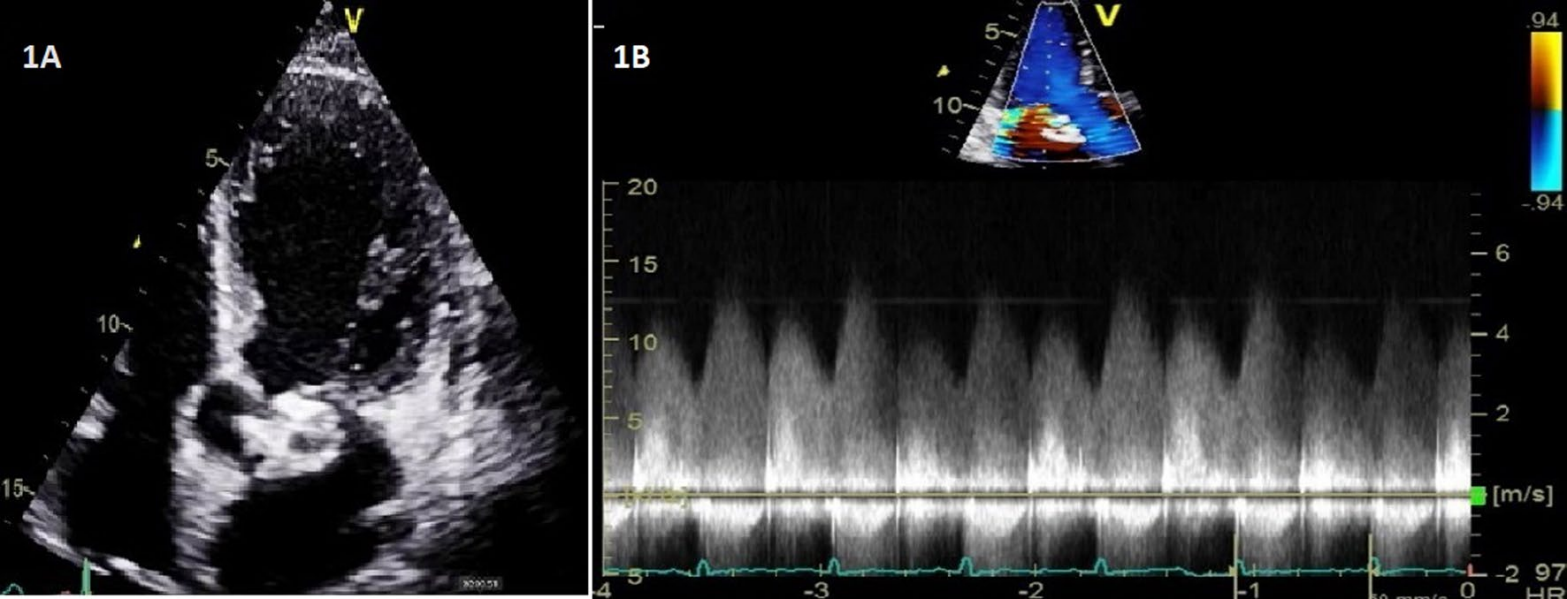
Transthoracic echocardiogram. A Apical five-chamber view on transthoracic echo showing vegetations above the aortic valve and an echo-free space in mitral-aortic intervalvular fibrosa consist with abscess. B Continuous-wave (CW) Doppler showing continuous flow due to fistula formation between the aorta and left atrium
